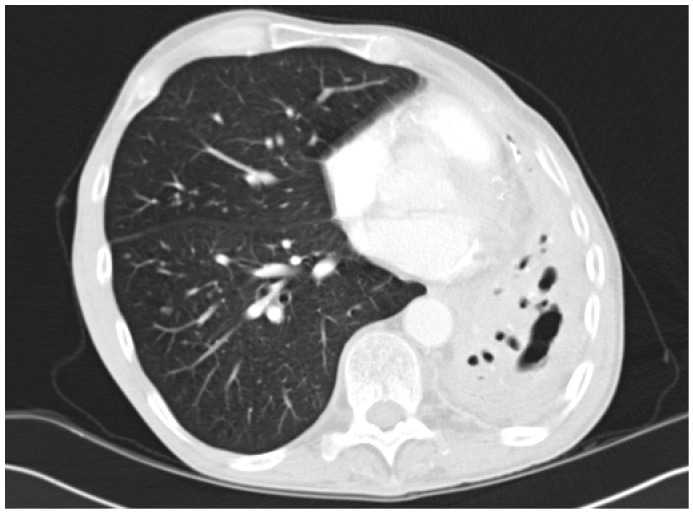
CT scan demonstrating CFPA with fibrosis and cavitation of the left lung resulting in significant volume loss in the left hemi-thorax.
